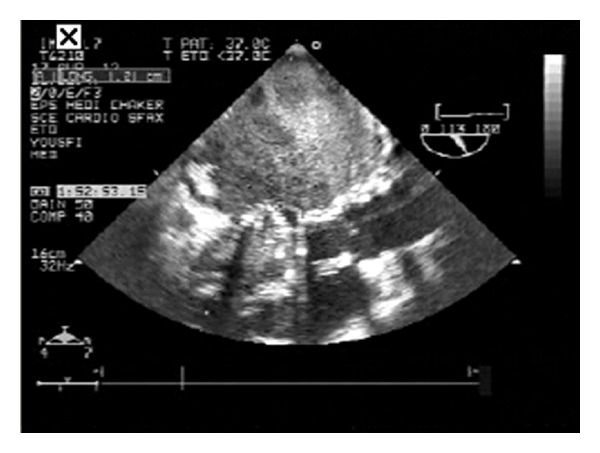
Transesophageal echocardiography (TEE) revealed vegetation on the anterior mitral annulus (1.2 ∗ 0.5 cm).
